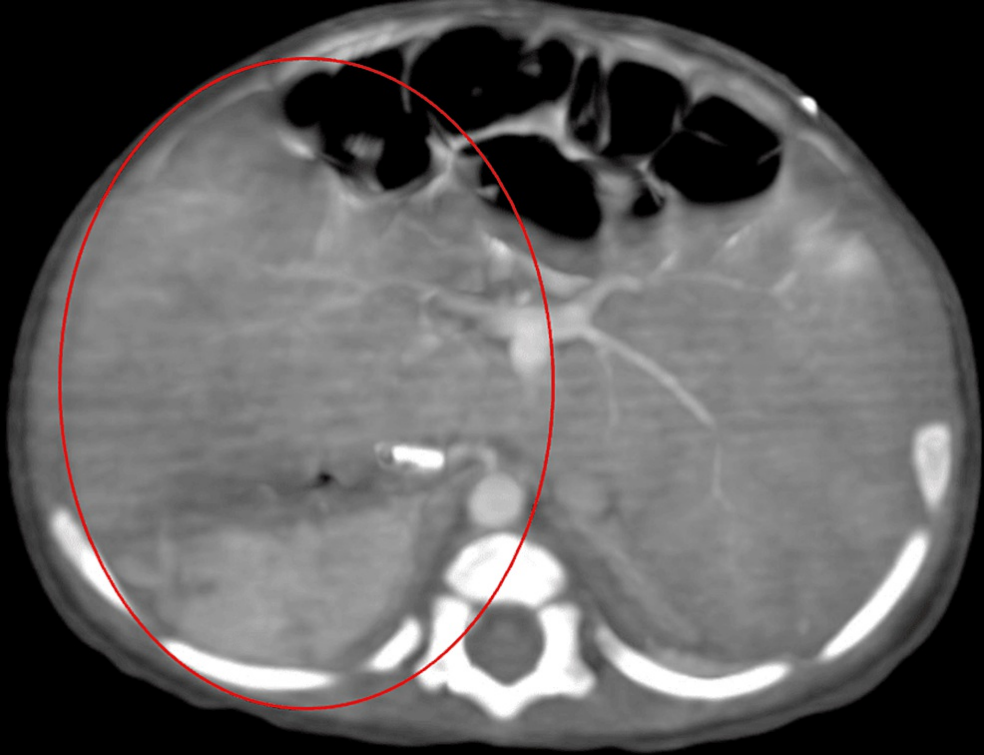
CT angiogram of the pulmonary arteries
